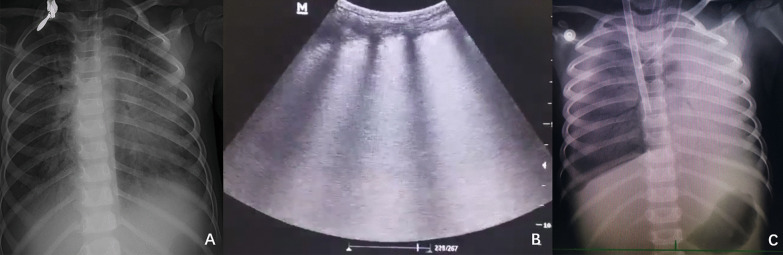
A The chest X-ray on the day before applying ECMO. B The lung ultrasound on the first day of applying ECMO. C The chest X-ray on the first day of applying ECMO
